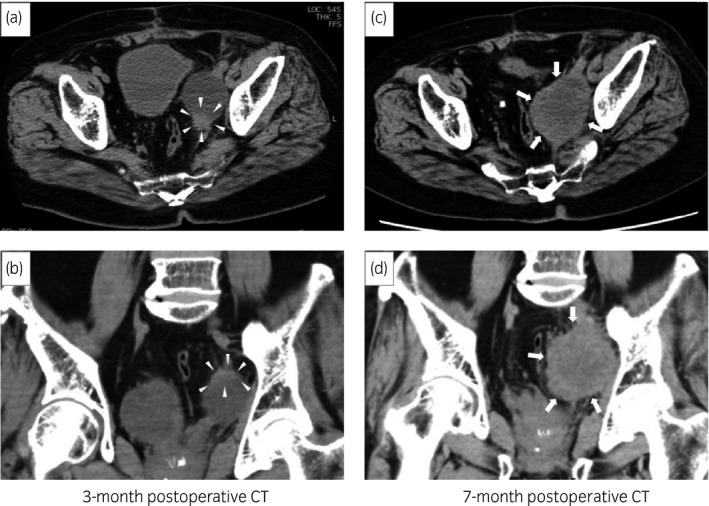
The postoperative 3‐month CT scan (a: axial view, b: coronal view) showed a lymphocele with a small solid component in the wall, in the left pelvic region. The coronal view showed the solid component area was distant from the UVJ. The postoperative 7‐month CT scan (c: axial view, d: coronal view) showed a solid mass had replaced the lymphocele in the left pelvic region.
